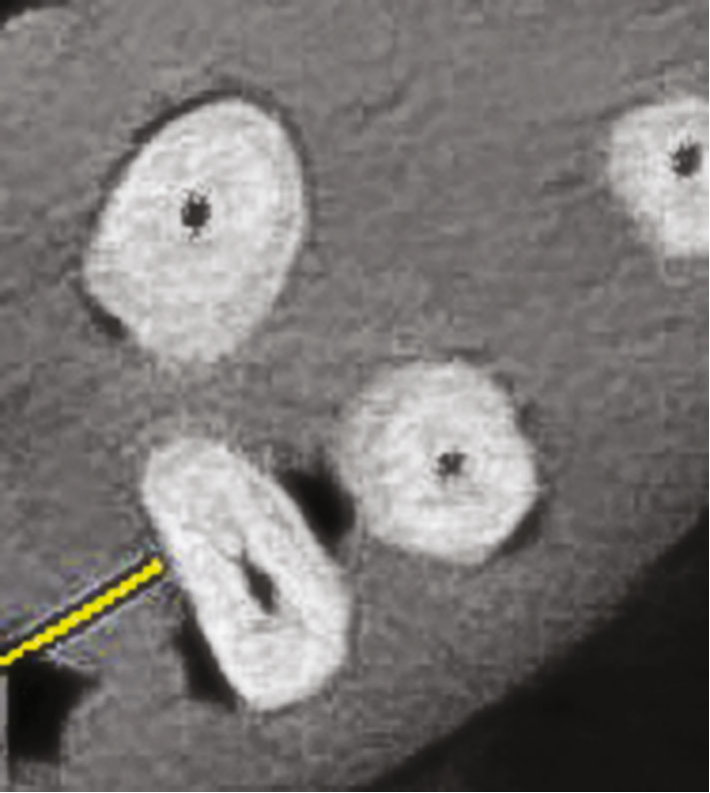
CBCT scan of the maxillary molar shown in Figure 3.
